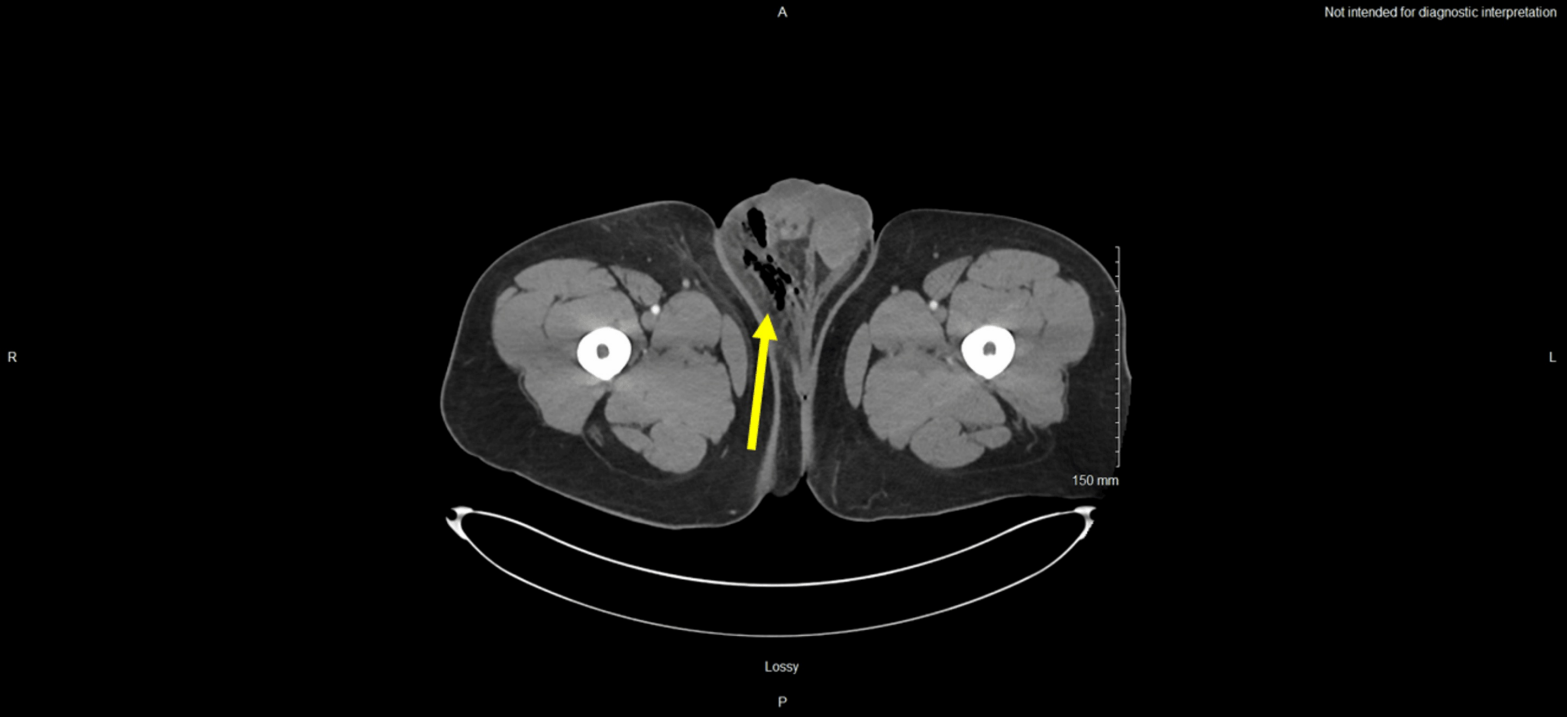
Axial view of the contrast-enhanced CT scan demonstrating soft tissue edema and subcutaneous emphysema involving the right hemiscrotum
